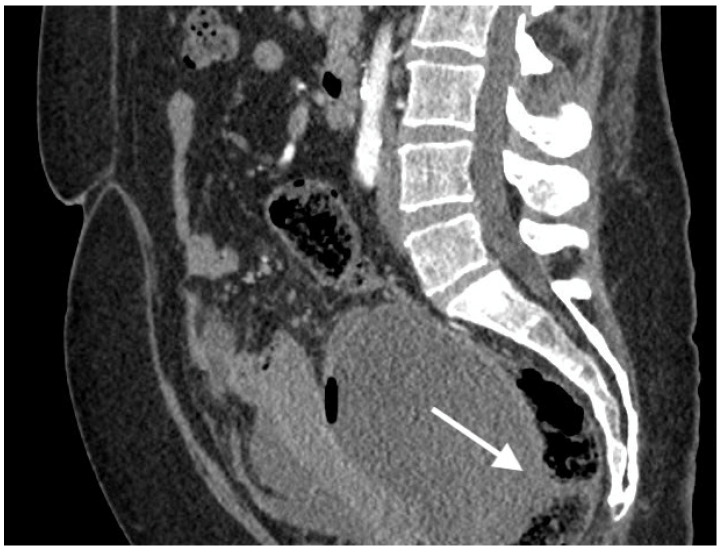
Images of a 57-year-old patient with high-grade serous ovarian cancer recurrence. Computed tomography shows large cystic-solid pelvic tumor with fluid/gas level and adjacent rectum as indirect sign of fistula.
